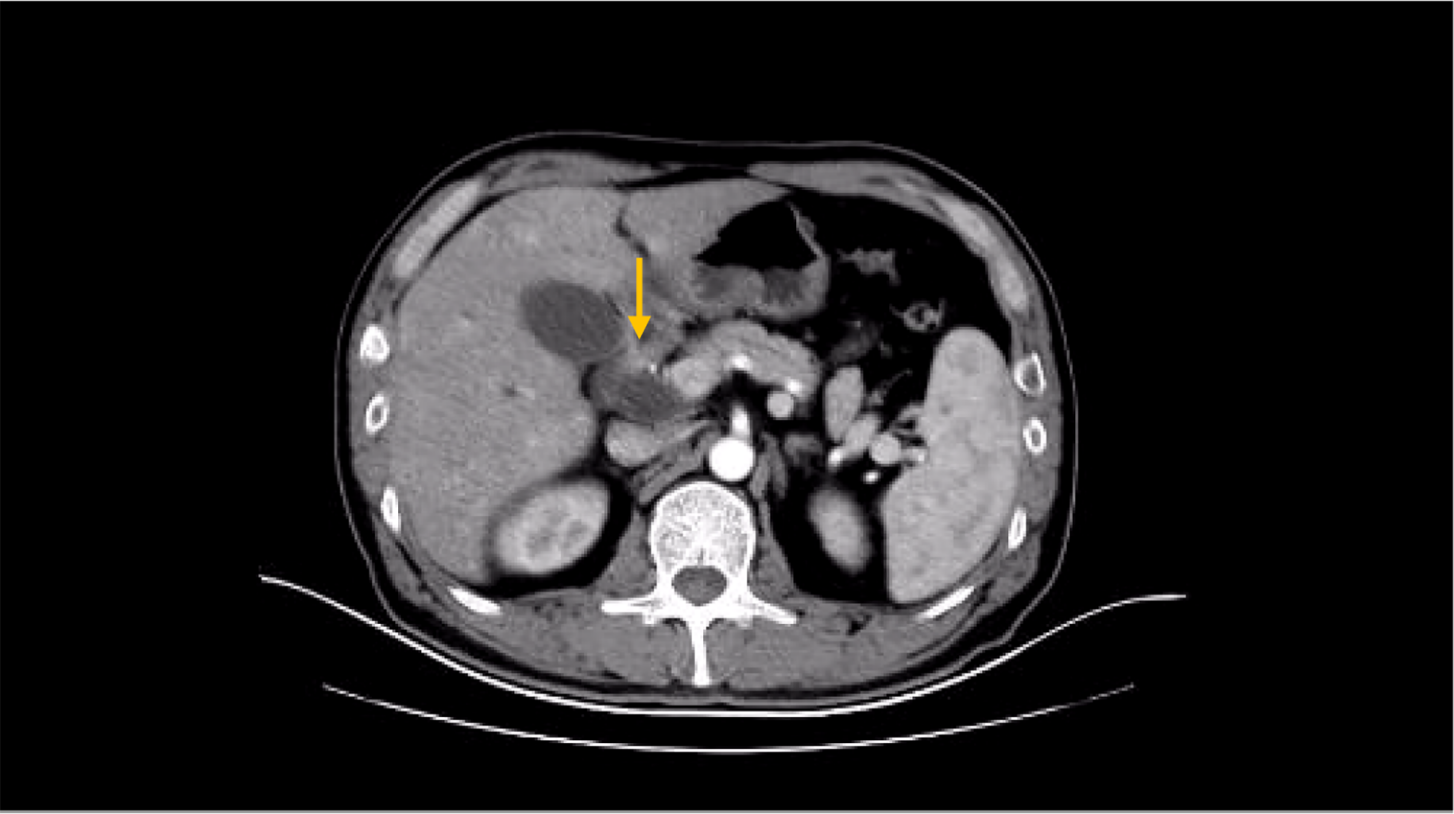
CT before operation (the tumor is located around the head of the pancreas).
